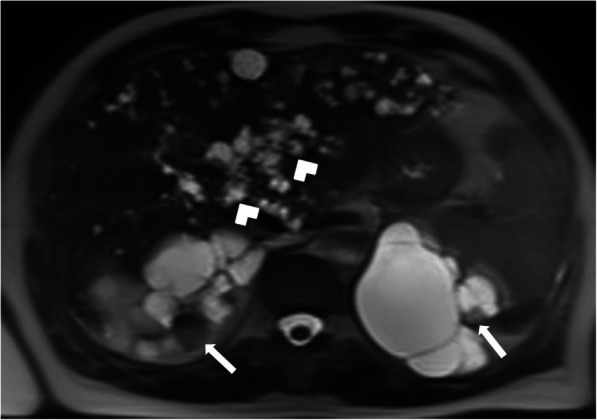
A 55-year-old female patient with known ADPKD and PLD. Axial plane T2W fat-saturated MR image shows multiple bilateral renal cysts with hemorrhagic cysts (arrows) and peribiliary cysts (arrowheads) in the liver
